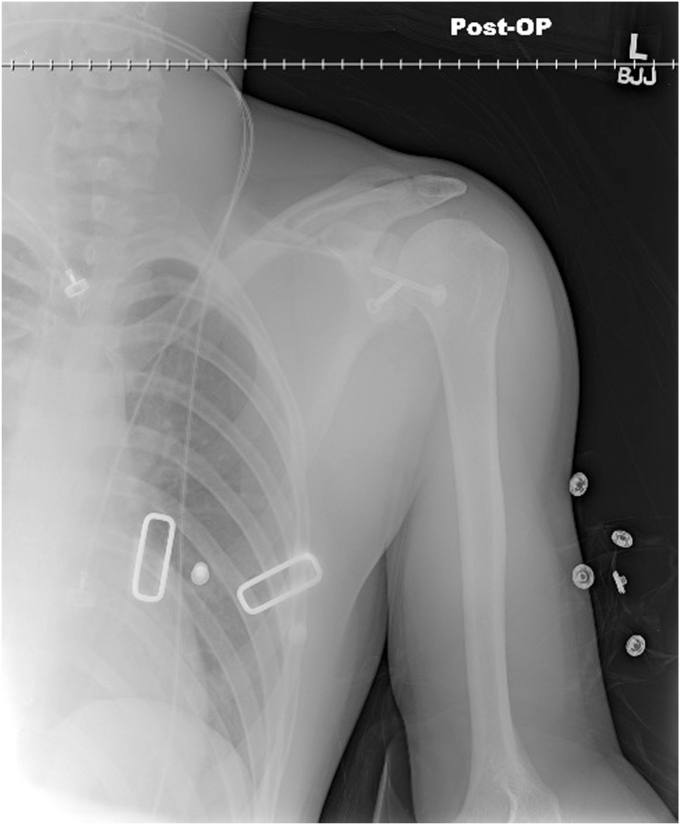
Anterior to posterior X-ray taken postoperatively after posterior glenoid reconstruction with scapular spine bone graft, capsular shift, and labral repair.
